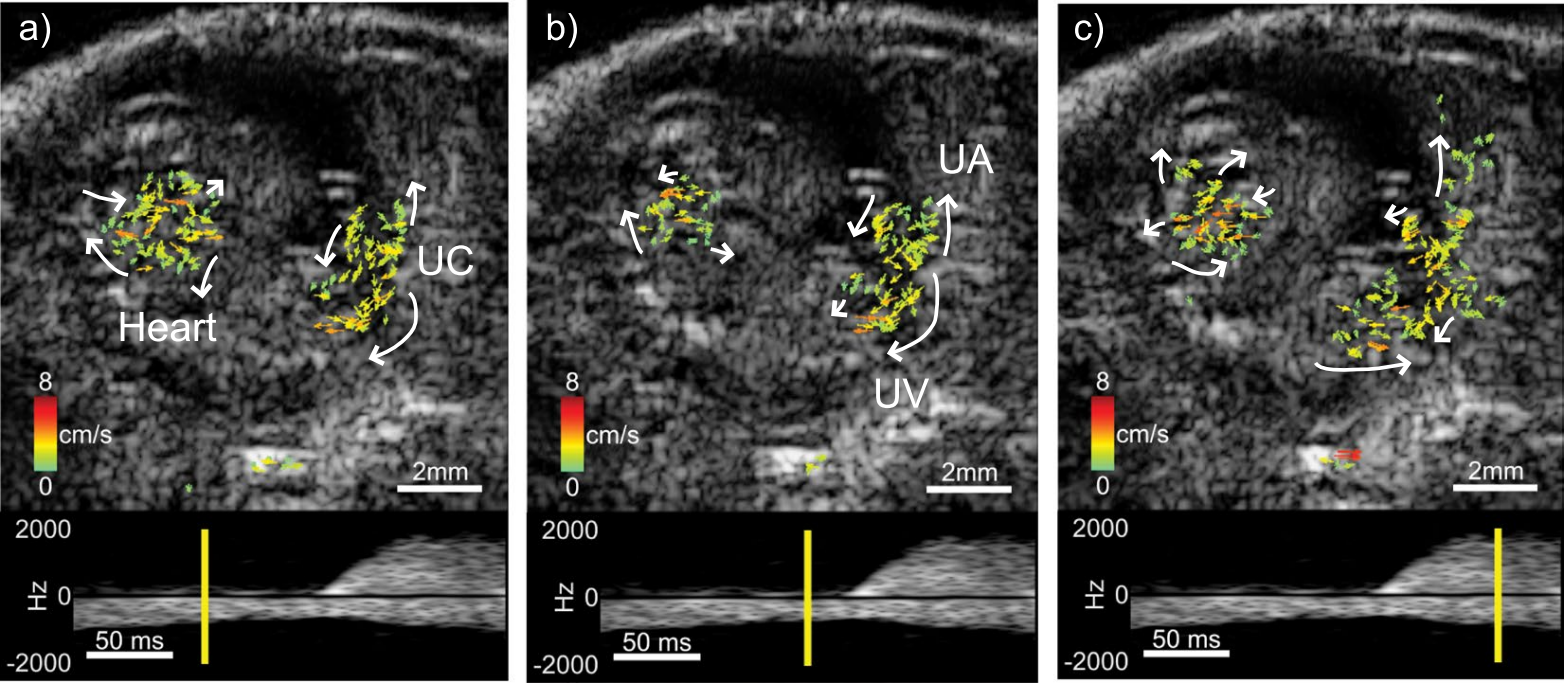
Three frames (76, 119 and 212 ms relative to start of spectrogram) from the vector-flow analysis of the data sequence of Fig. 5. The Doppler spectrogram represents the same region of the umbilical cord (UC) as was indicated in Fig. 5a. The yellow line in the spectrogram indicates the slow-time point for which the data is displayed. (a) Early systole with steady flow in umbilical vein. (b) Late diastole to early systole where flow in heart is minimal. (c) Flow in heart increasing during diastole. The white arrows highlight the general direction of local flow vectors in each frame. Flow vectors point in the direction of flow and their color and length scale with the vector magnitude. UA: Umbilical artery, UV: Umbilical vein. The full image sequence is available as Movie 4.
